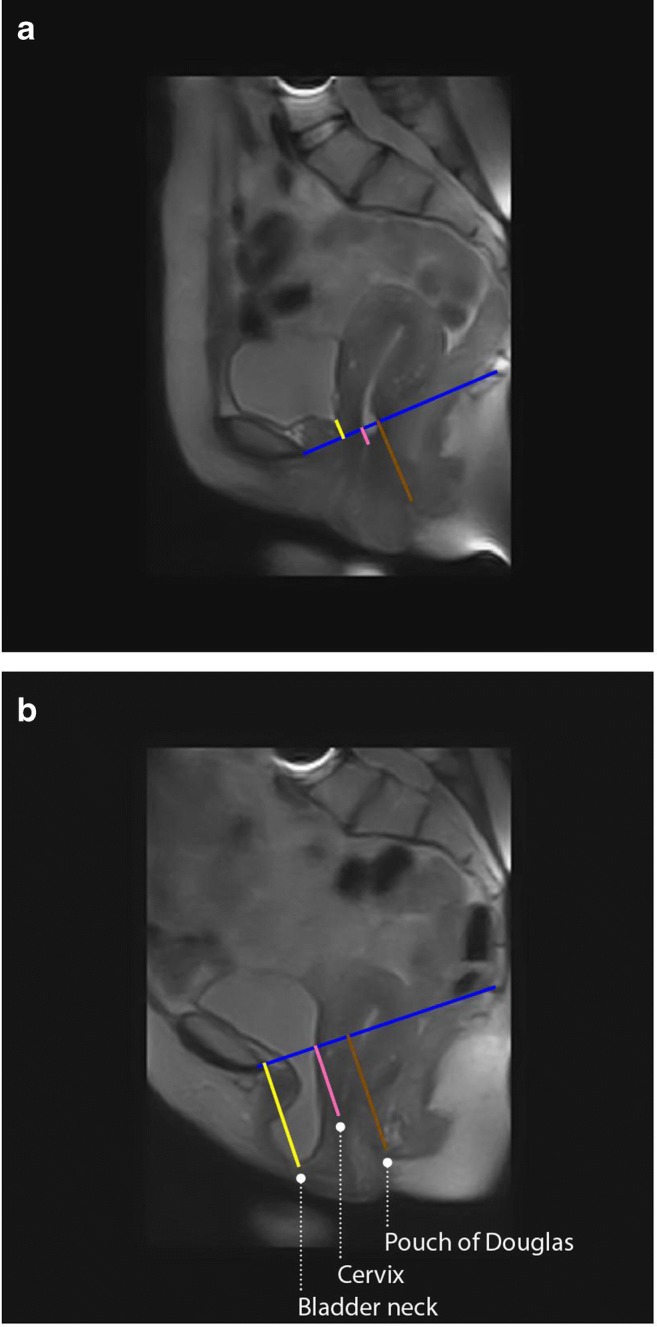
Sagittal MRI scan of a patient in a the supine–rest and b the upright–rest position. The *blue line* represents the pubococcygeal line (PCL). The *yellow*, *pink*, and *brown lines* visualize the distances of the PCL from the bladder neck, cervix, and pouch of Douglas respectively
